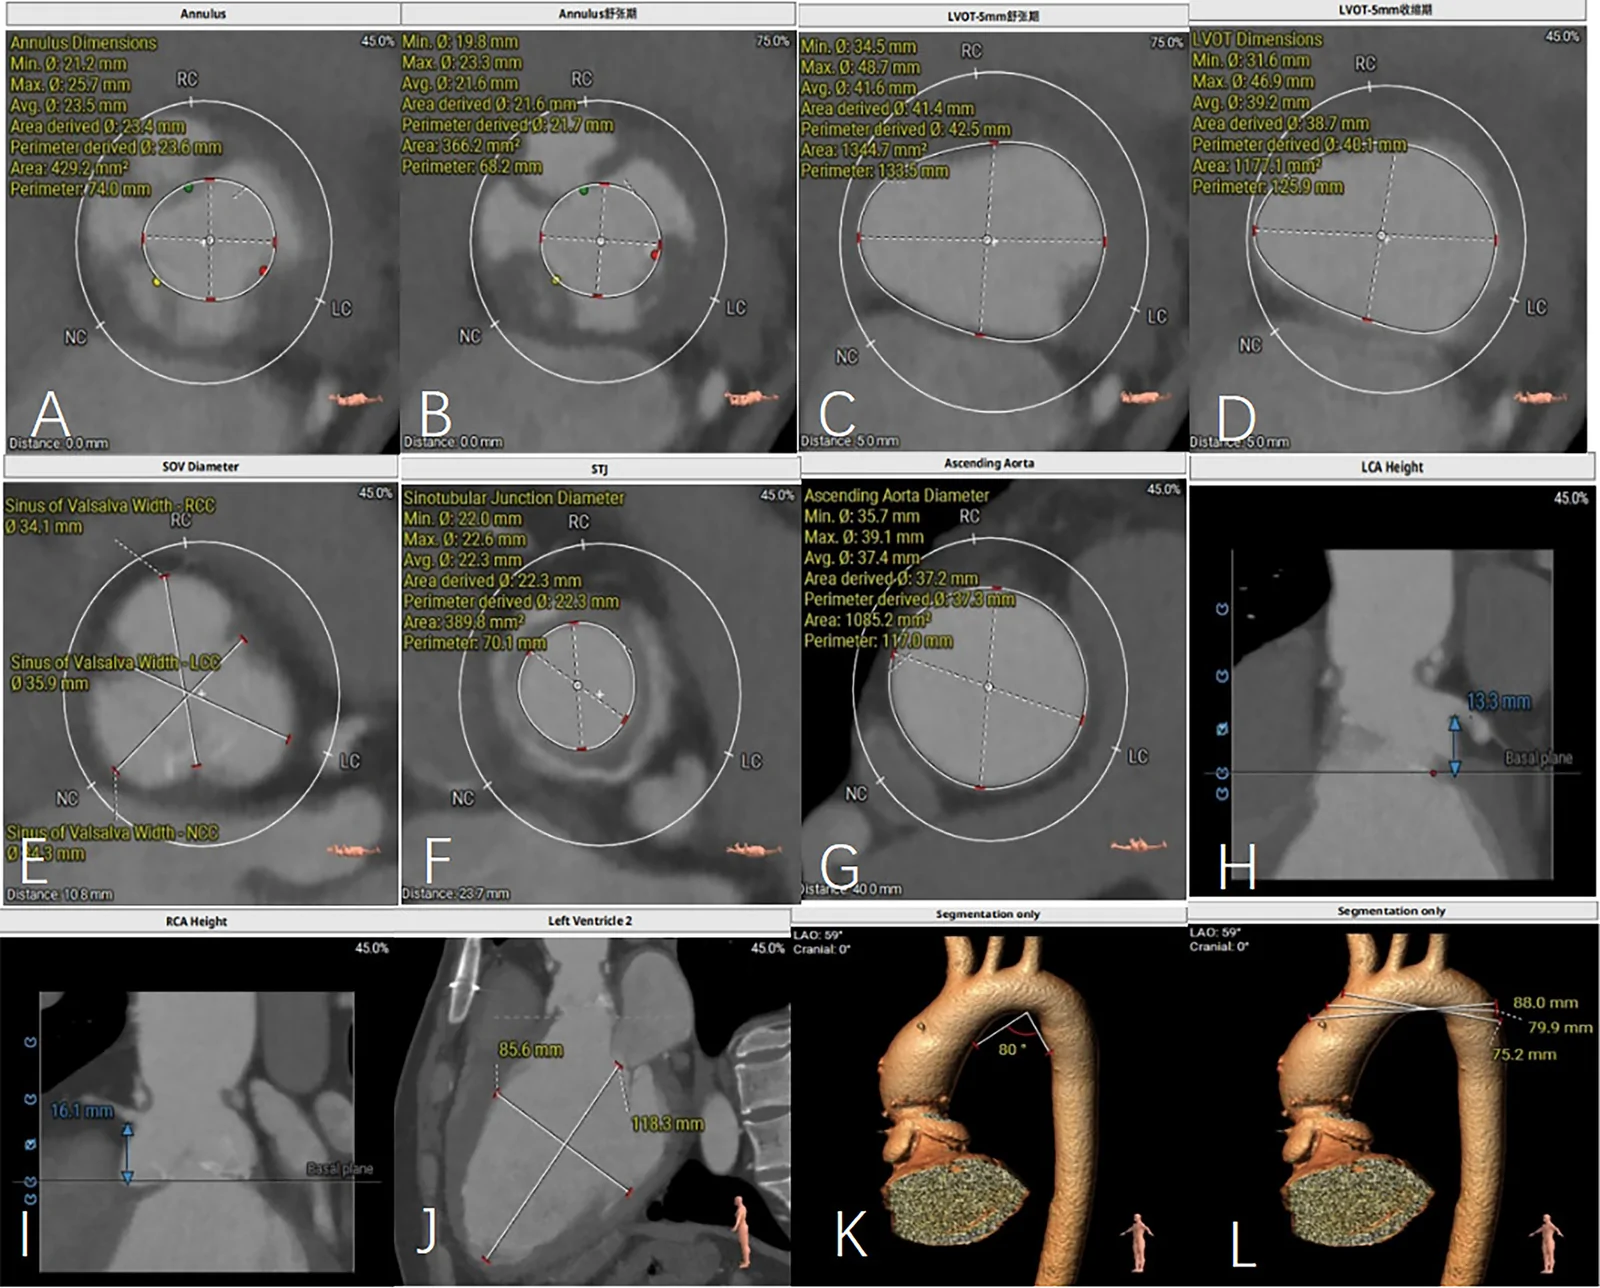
Preoperative CTA assessment. (A) Annular diameter during systole 29.9 mm; (B) Annular diameter during diastole 29.3 mm; (C) Diastolic diameter of the left ventricular outflow tract 42.5 mm; (D) Systolic diameter of the left ventricular outflow tract 40.1 mm; (E) Sinus of Valsalva diameters 34.1 mm, 35.9 mm, 34.3 mm; (F) Sinotubular junction diameter 22.3 mm; (G) Ascending aorta diameter 37.3 mm; (H) Height of the left coronary artery orifice 13.3 mm; (I) Height of the right coronary artery orifice 16.1 mm; (J) Left ventricular longitudinal diameter 118.3 mm, left ventricular transverse diameter 85.6 mm; (K) Aortic arch angle 80 degrees; (L) Aortic arch distance 88.0 mm.
